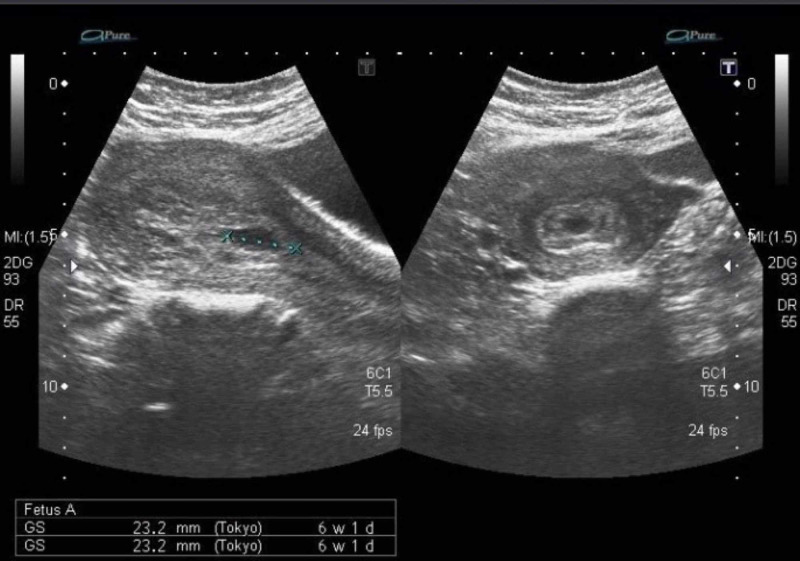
Pelvic ultrasound showing thick endometrium
